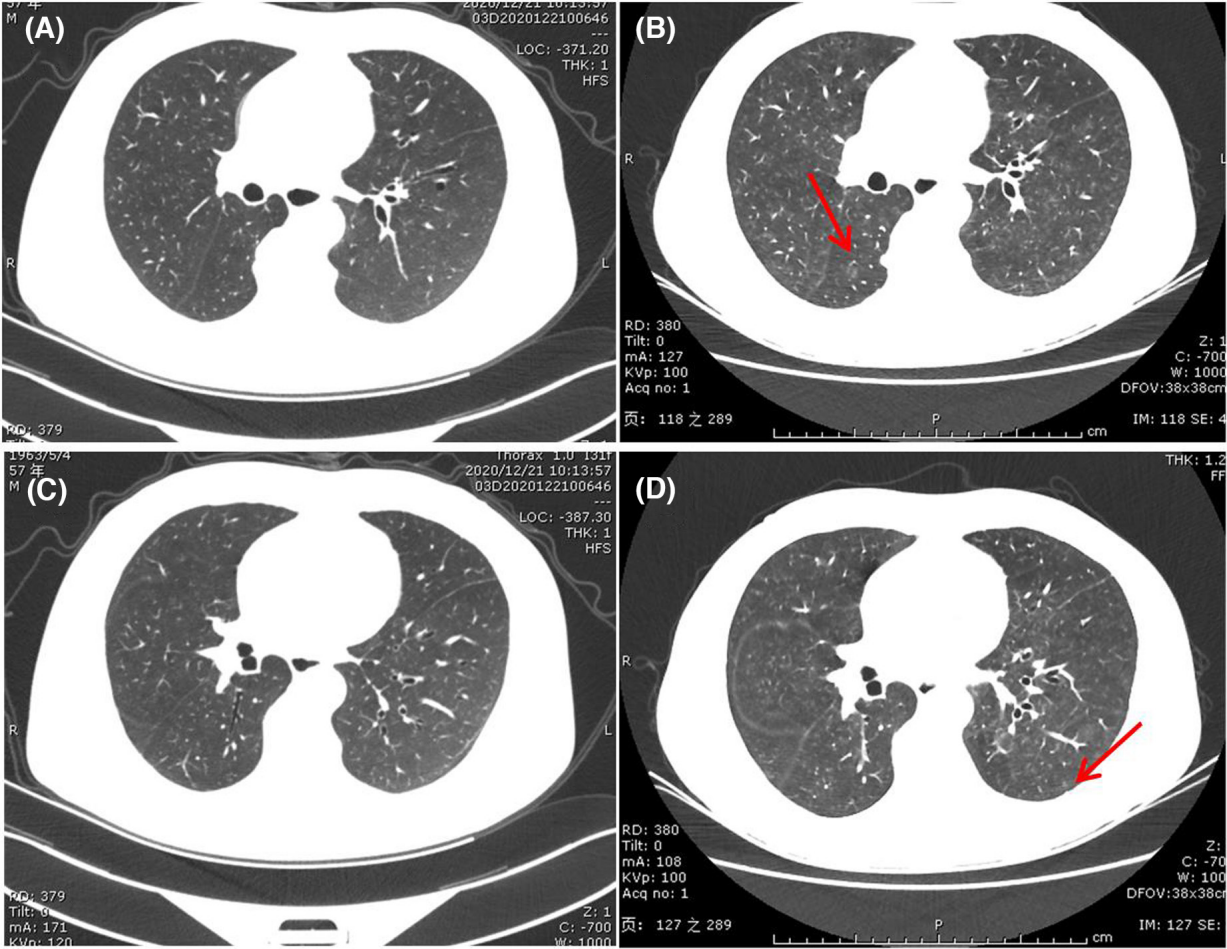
HP pattern in a 58‐year‐old man who underwent nivolumab therapy for stage IV lung adenocarcinoma. (A and C) A baseline chest CT image before starting immunotherapy (not shown) showed no airspace abnormalities. (B and D) Axial chest CT images obtained 5 months after starting nivolumab therapy shows diffuse centrilobular ground‐glass nodules (arrows).
